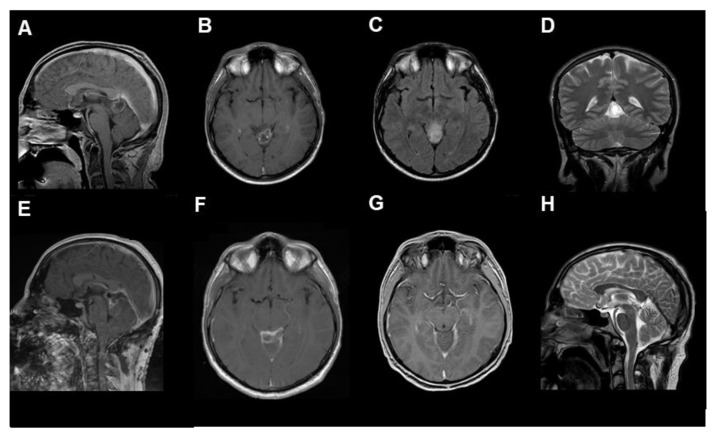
The radiological findings of RGNT in the pineal region ((A–D) preoperative, (A,B): T1-weighted with contrast medium, (C): T2 flair-weighted, (D): T2, (E,F): postoperative, T1-weighted with contrast medium, (G,H): 3-months follow-up, (G): T1-weighted with contrast medium, (H): T2-weighted), from the first case, a 23-year-old male patient who presented with chronic headache.
